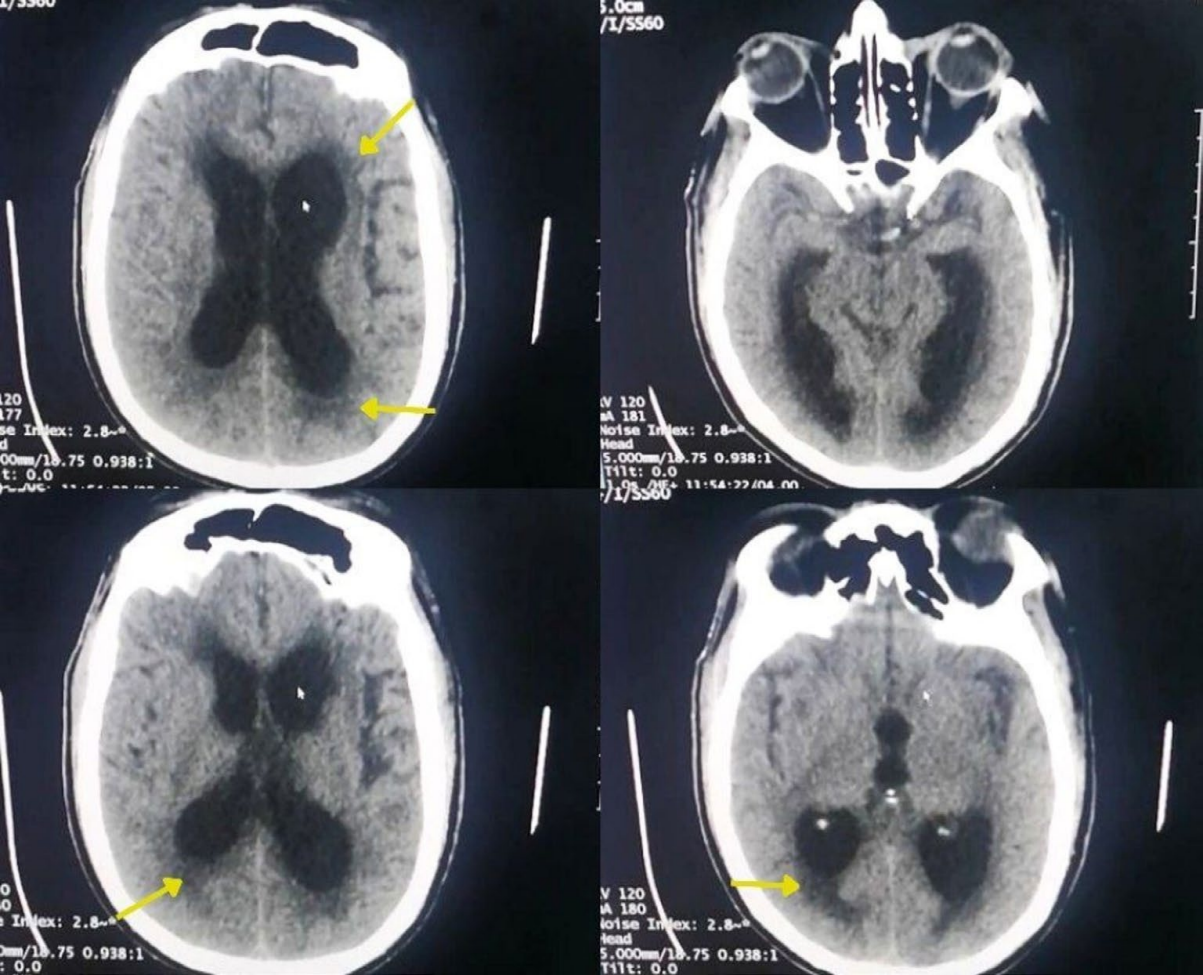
Axial CT without contrast reveals enlarged lateral and third ventricles, along with the effacement of sulci and widening of the Sylvian fissure. Additionally, periventricular hypodensities are noted (indicated by yellow arrows).
